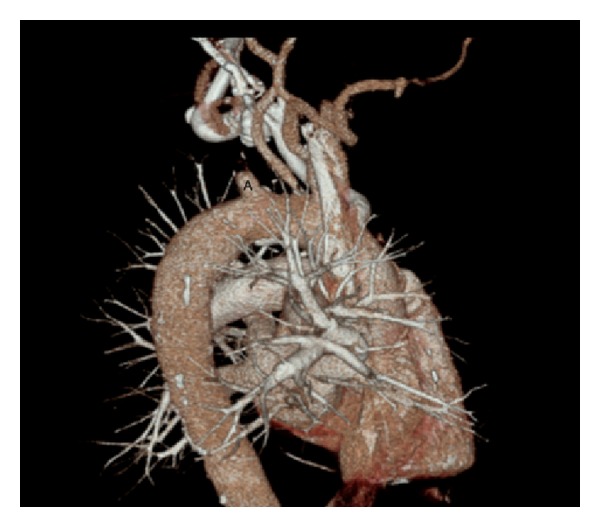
CTA of the chest with complete occlusion of left subclavian artery (A).
